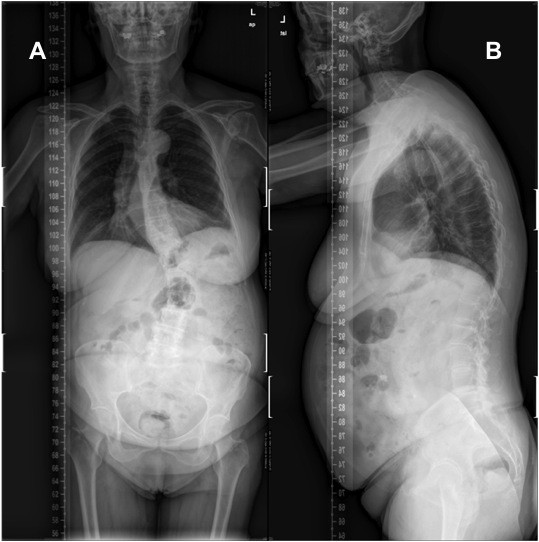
Preoperative p-a (A) and lateral (B) x-rays demonstrating degenerative lumbar scoliosis with anterolateral lystesis at L2-L3-L4 and L5.
